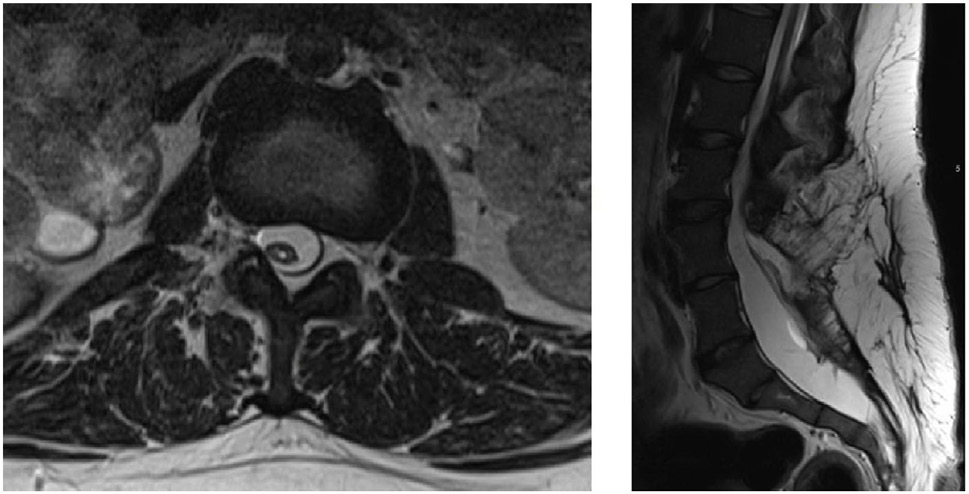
Axial and sagittal T2 lumbar MRI showing postsurgical changes from L3-L5 with neural elements present to the sacrum with an associated syrinx.
